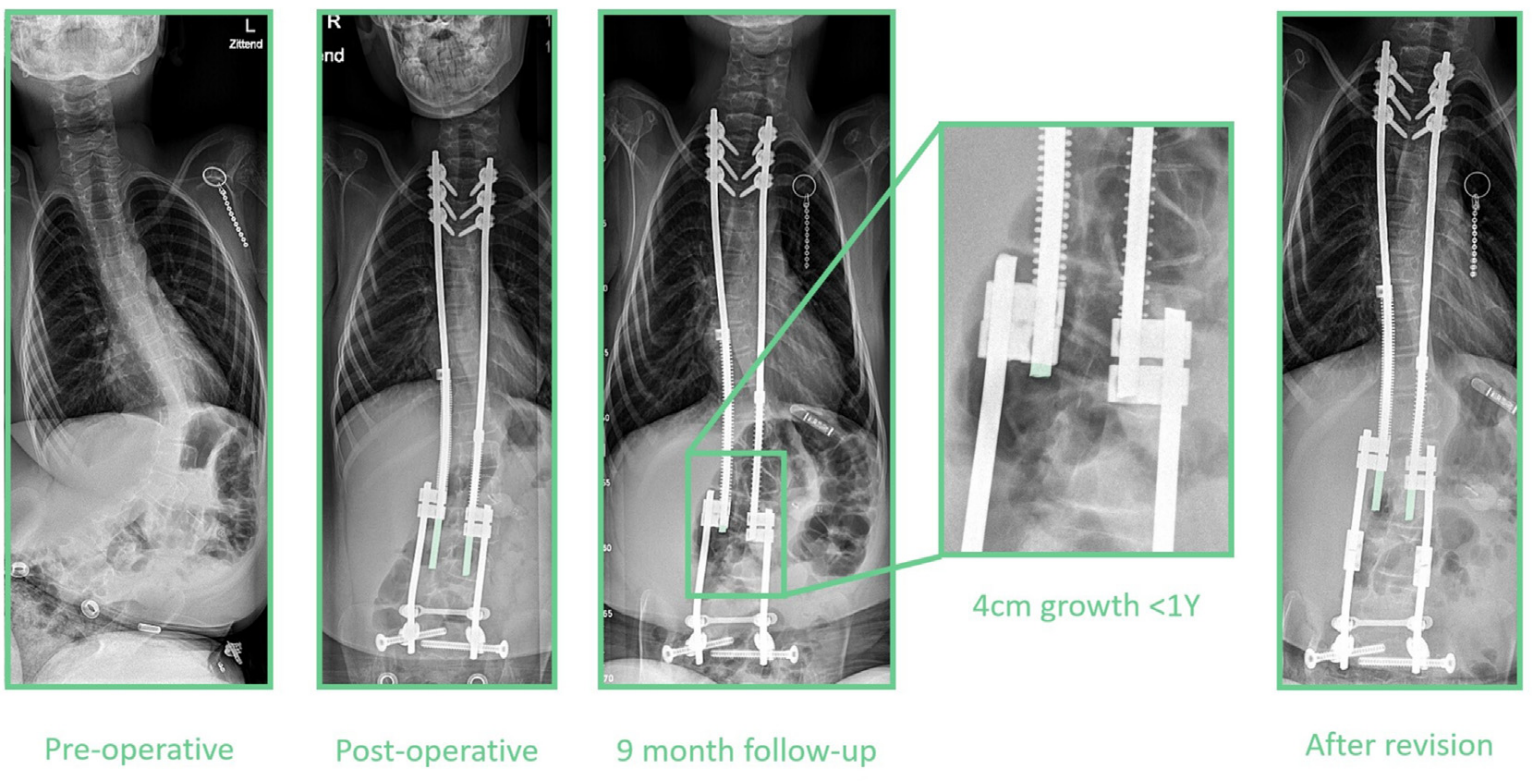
SDS complication. Example of an SDS patient that showed almost complete implant expansion within 1 year. Note the initial lengthening between insertion and the first radiograph of 20 mm. As there was still at least 3 years of expected spinal growth, the choice was made to perform a re-operation in which the springs were re-tensioned and the distal anchor rod construct was lengthened. The colored segment of the sliding rod denotes how much implant growth is left.
